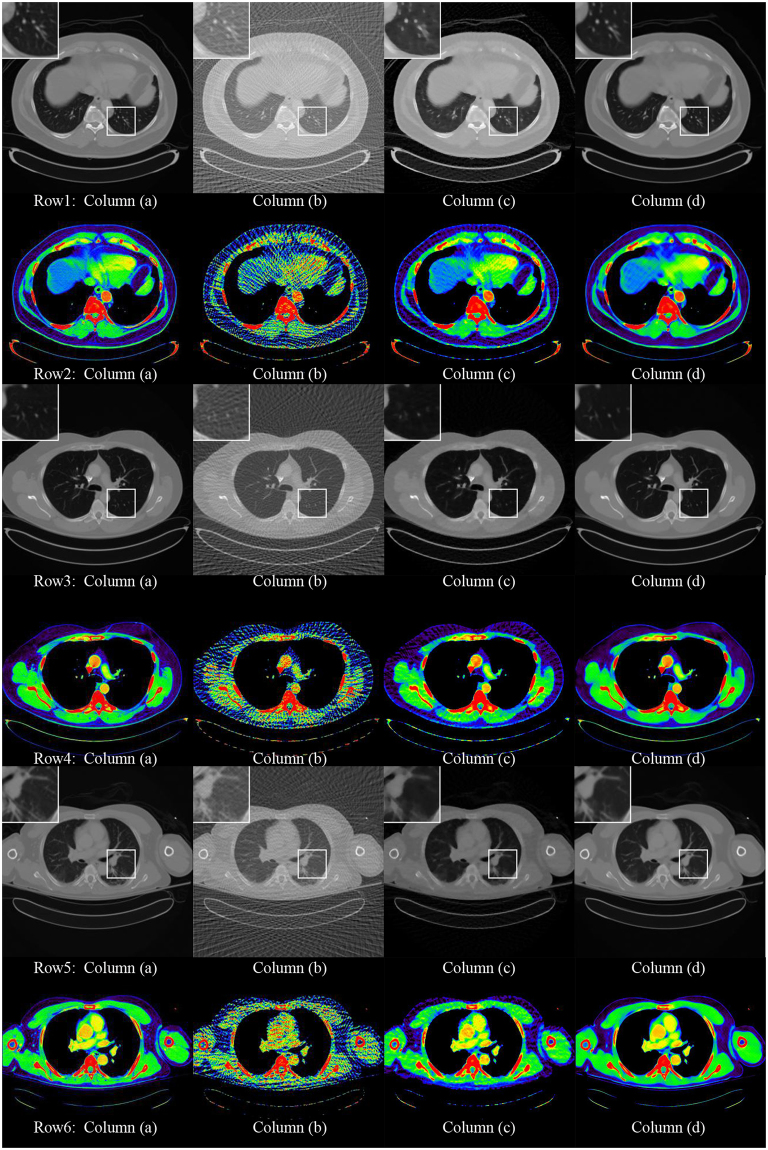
The 512*512 CT images reconstructed by 120 projection views. Column (a) shows the reconstruction by the FBP method from the full projection views. Column (b) shows the reconstruction by the FBP method from the sparse projection views. Column (c) shows the reconstruction by the ADS-POCS method from the sparse projection views. Column (d) shows the reconstruction by the proposed method. All images display in the same window at row 1, 3 and 5. In order to compare the CT value with different reconstruction method, we use 2D atlas to display. The result is shown in row 2, 4 and 6.
